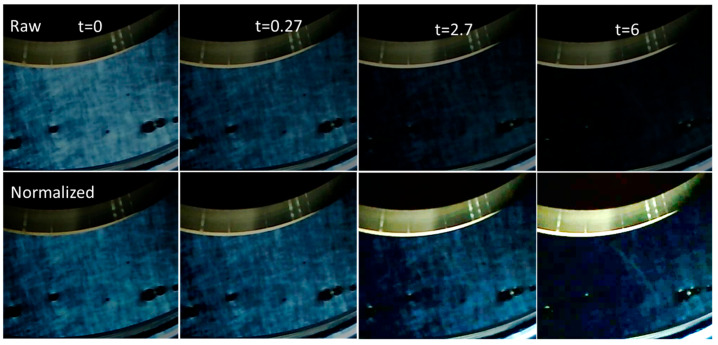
Example of birefringence relaxation after stopping shear (10 s^−1^) for CT-DNA/TE solution at 10 mg/mL. Top: Images extracted from the movies. Bottom sequence: The same images, but with the intensity normalized to the second image to obtain the best contrast. Measurements were performed at 20 °C.
